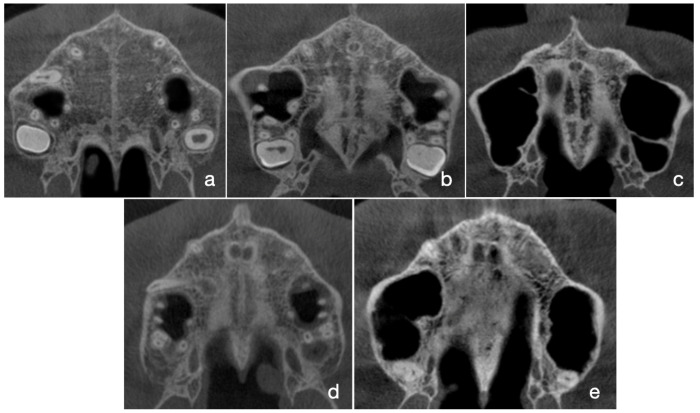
Representative CBCT images of the five maturation stages. At stage A, only a high-density line in visible (a). At stage B, a scalloped high-density line can be visualized (b). At stage C, two parallel, scalloped, high-density lines are visible, that are separated by some small, low-density areas (c). At stage D, two scalloped lines can be seen; however these lines cannot be visualized in the palatine bone, and the parasutural palatine bone density is higher when compared with the parasutural maxillary bone density (d). At stage E, the MPS cannot be visualized as the fusion has occurred, and the density of the bone is homogeneous (e).
